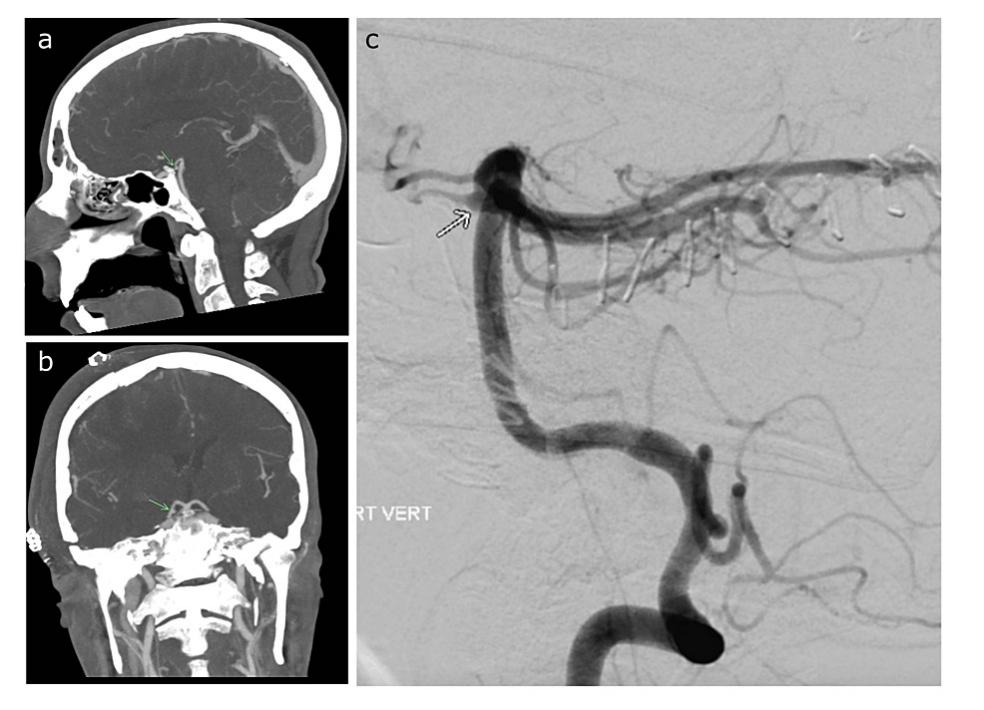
CT angiogram of the head 8.0 mm maximum intensity projections in (a) the sagittal and (b) the coronal planes demonstrating a dilation concerning for aneurysm arising from the right PCA (arrow); (c) DSA of the right vertebral artery in lateral projection demonstrating posterior communicating artery infundibulum arising from the proximal (P1-segment) posterior cerebral artery (arrow) PCA: posterior cerebral artery; DSA: digital subtraction angiography
